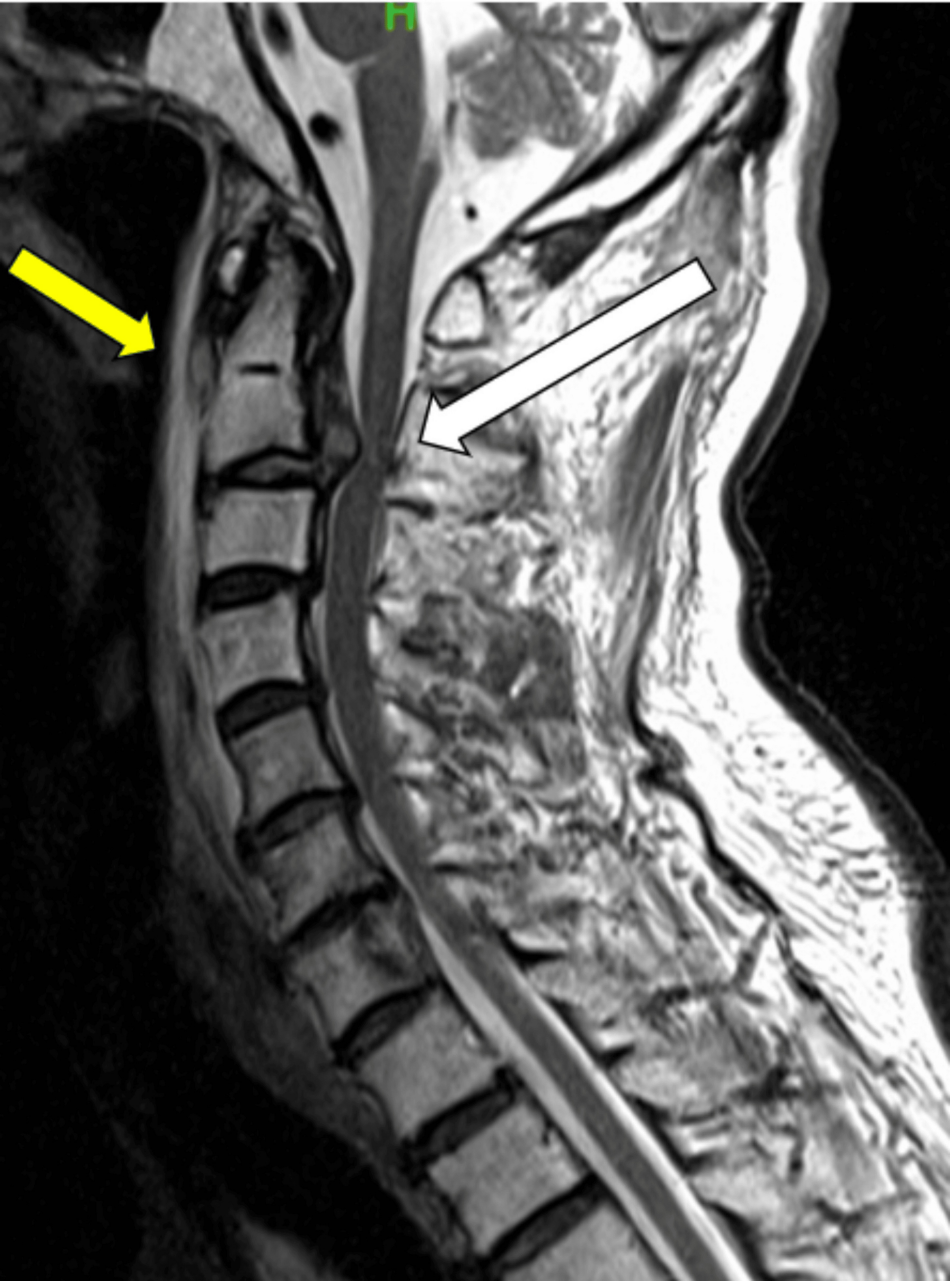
Sagittal T2-weighted MRI revealing a C2/3 disc herniation with cord compression (white arrow) and the pre-vertebral hematoma (yellow arrow) on the anterior aspect of the C2-5 vertebral body upon patient admission.
